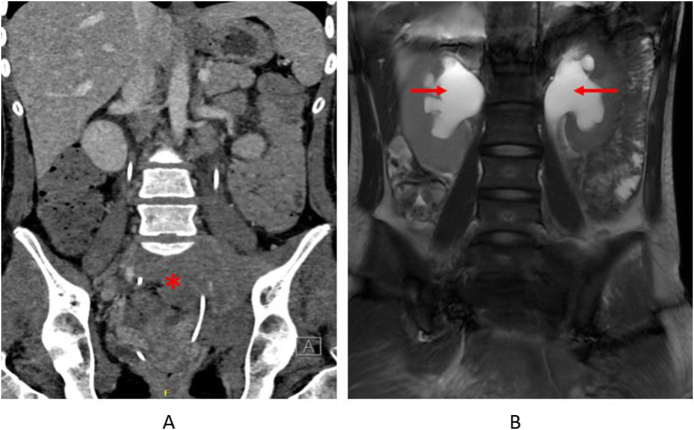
Imaging findings in one of the pediatric patients. (A) Abdominal CT scan showing a pelvic mass (asterisk). (B) MRI demonstrating bilateral hydronephrosis (arrow).
